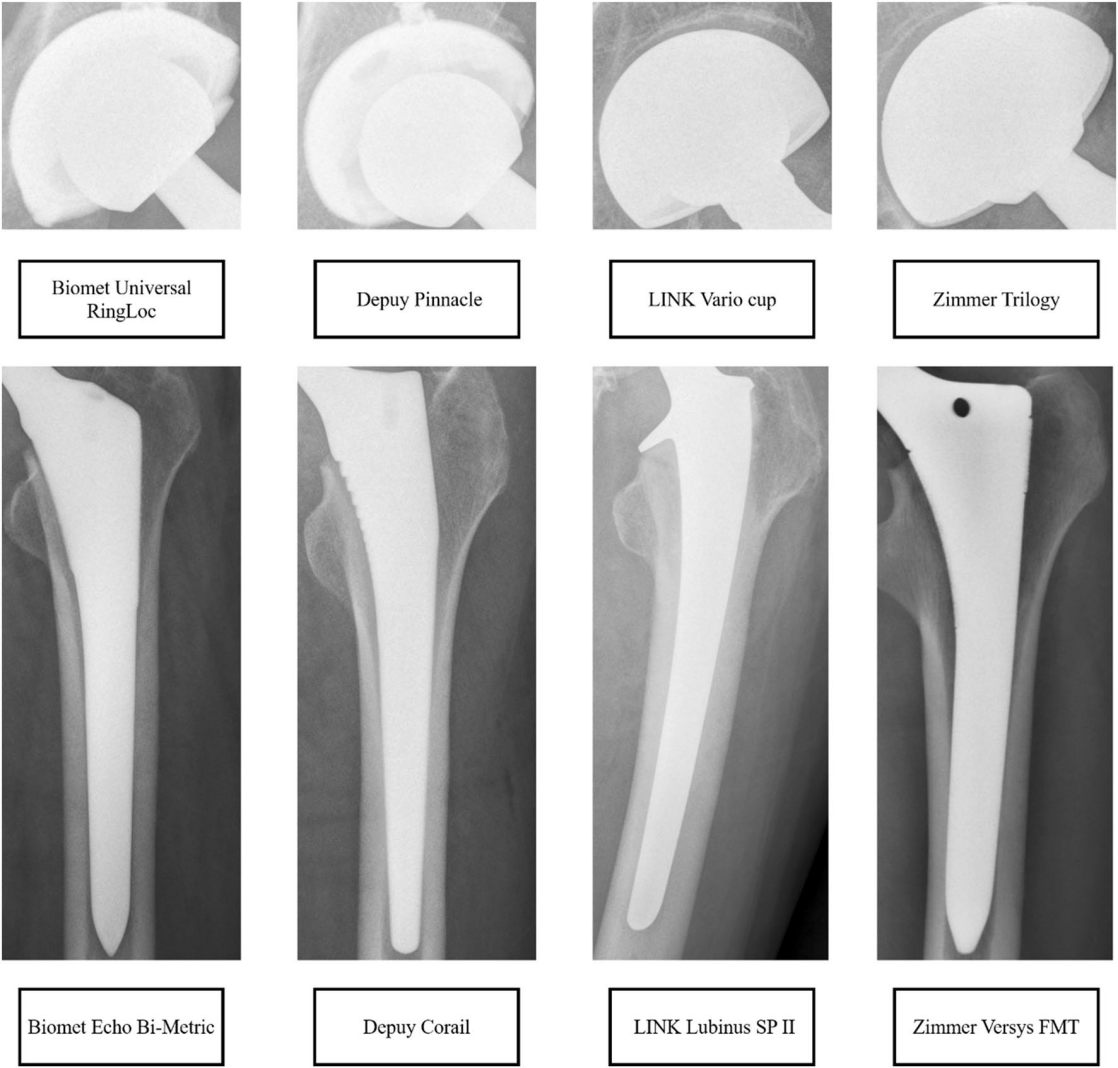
Demonstrated an example of cup and stem radiographs of each included implant design.
